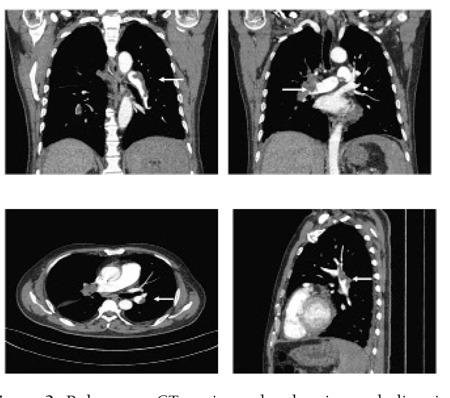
Pulmonary CT angiography showing embolism inthe pulmonary arteries and their branches (arrow)
